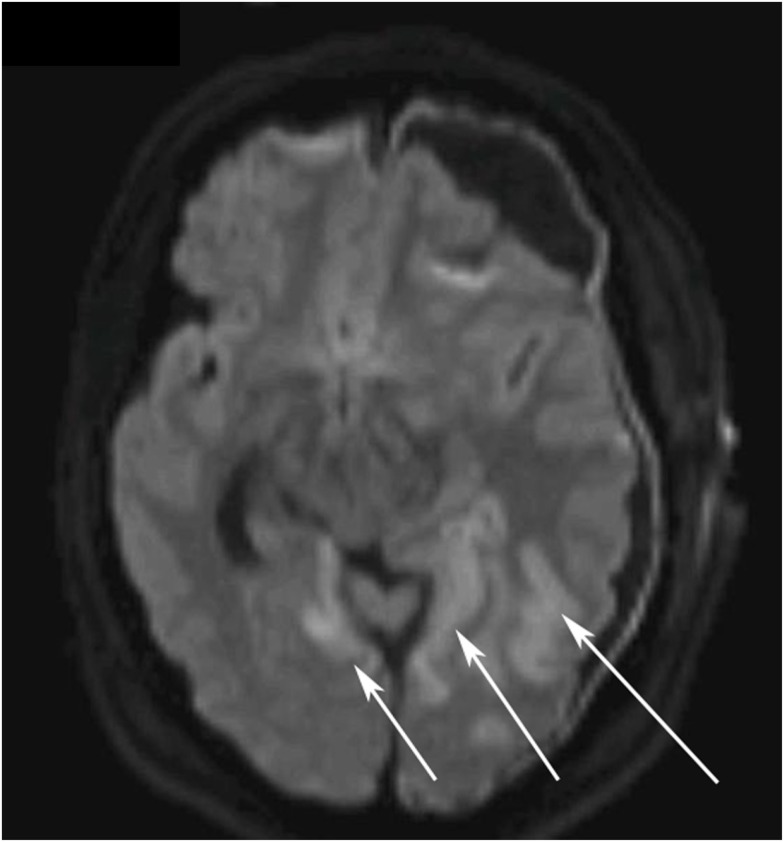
Diffusion weighted magnetic resonance imaging (DWI MRI) of the brain on day 5. The white arrows highlight the ischemic regions in the left and right occipital lobes.
